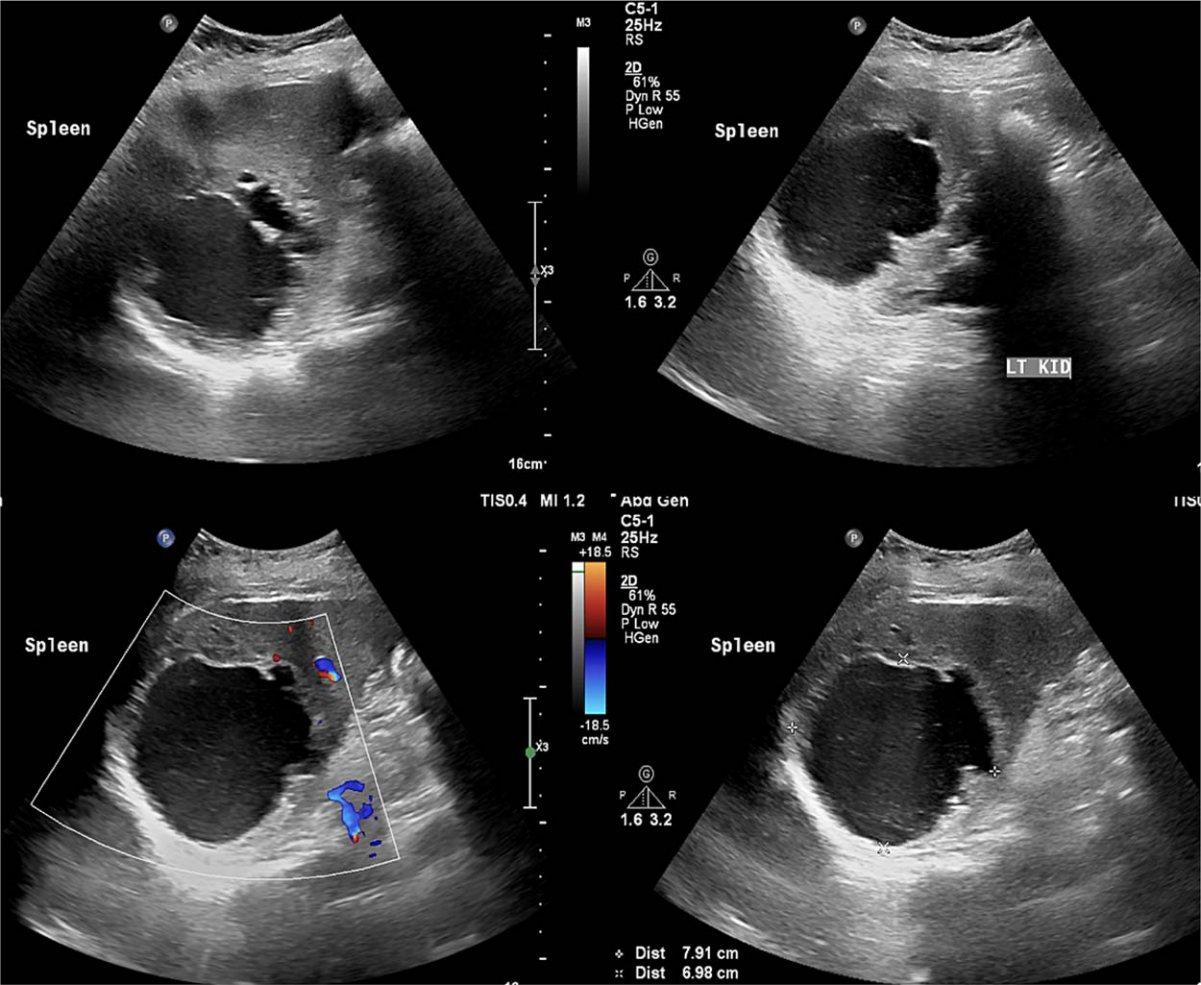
Abdomen ultrasound revealed well-defined multilocular cystic lesion with internal echoes in the spleen, measuring ~7.9 × 6.9 cm.
